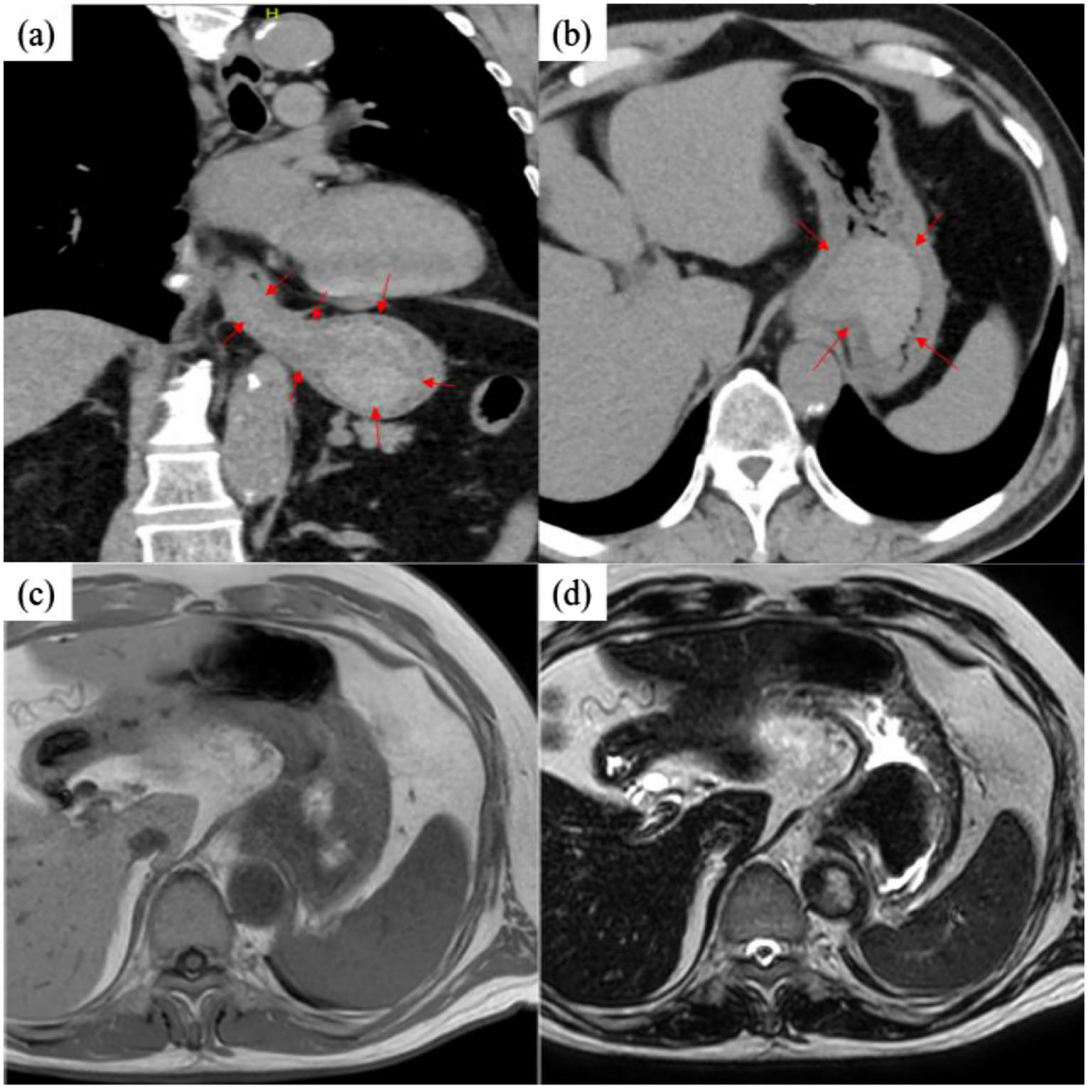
(a) Admission plain computed tomography scan showed a continuous hyperabsorptive zone from the thoracic esophagus to the stomach. (b) In the stomach, a clearly demarcated hyperabsorptive zone was observed from the fundus to the upper gastric body, which was considered to be a hematoma. (c) Magnetic resonance imaging on day 2 showed a pale high signal area in the stomach on T1‐weighted images. (d) T2‐weighted images also showed a low signal area, leading to the diagnosis of acute to subacute submucosal hematoma.
